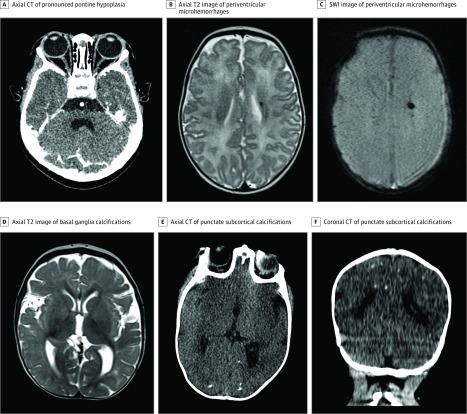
Abnormal Neuroimaging Findings in Infants Who Were Asymptomatic, Had Mild to Moderate Zika Virus Infection at Birth, or Had Normal Neurologic Evaluation Findings at Birth A, Axial contrast-enhanced computed tomography (CT) image through the brainstem demonstrates pronounced pontine hypoplasia. B, Axial T2 image demonstrates punctate susceptibility artifact along the margin of the left lateral ventricle consistent with periventricular microhemorrhages. C, Susceptibility-weighted image (SWI) demonstrates punctate susceptibility artifact along the margin of the left lateral ventricle consistent with periventricular microhemorrhages. D, Axial T2 image demonstrates punctate susceptibility artifact in the bilateral basal ganglia consistent with basal ganglia calcifications. E, Axial CT without contrast demonstrates punctate subcortical calcifications in the occipital lobes. F, Coronal CT without contrast demonstrates multiple punctate subcortical calcifications as well as a punctate periventricular calcification along the superior margin of the right lateral ventricle.
